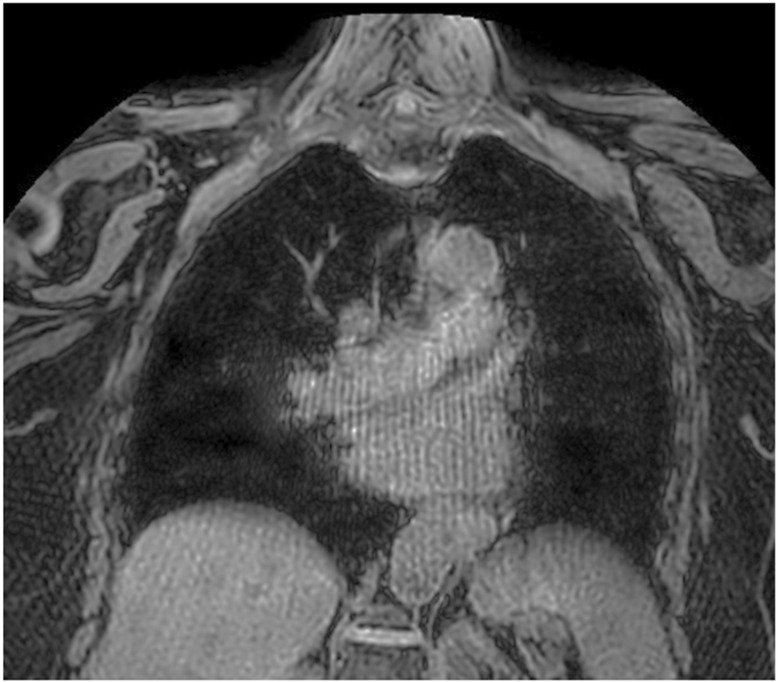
Unenhanced radial T1-weighted MRI sequence showing streak artifacts in the coronal plane.
